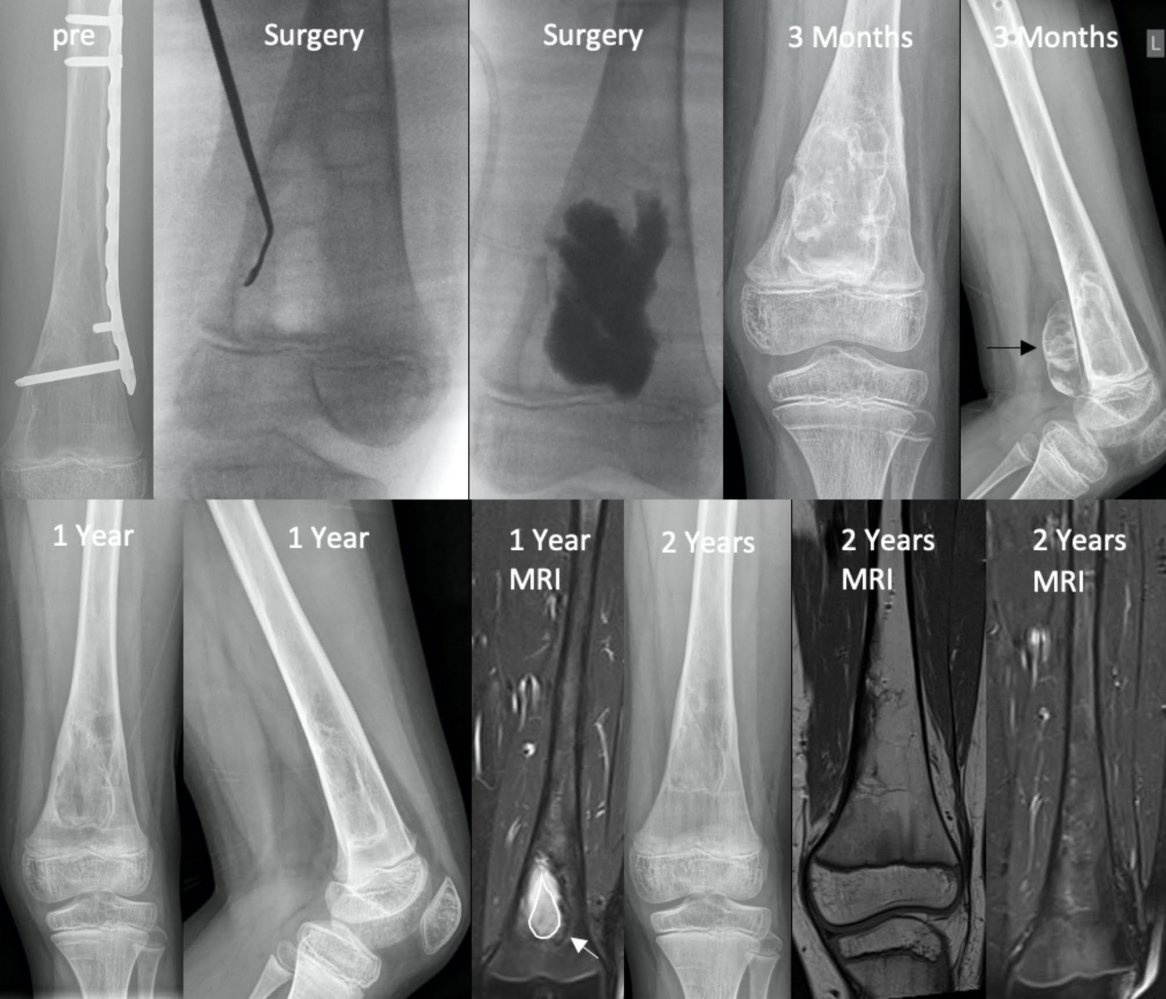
A 10-year-old male patient who suffered from a pathologic fracture due to a unicameral bone cyst and consequently received open curettage, filling with allograft bone chips and osteosynthesis. (Pre) Recurrence was visible two years after surgery, which led to osteosynthesis removal, open curettage and filling with a BCBS. (Surgery) On early postoperative X-rays, residual BCBS in the popliteal soft tissue (black arrow) could be observed, which gradually vanished by one year after surgery. On MRI one year after surgery, a typical inhomogeneous T2 hyperintense transformation zone (white arrow) and a teardrop-shaped filling core (white circle) could be seen, which faded with bony integration on follow-up MRI two years after the index procedure
